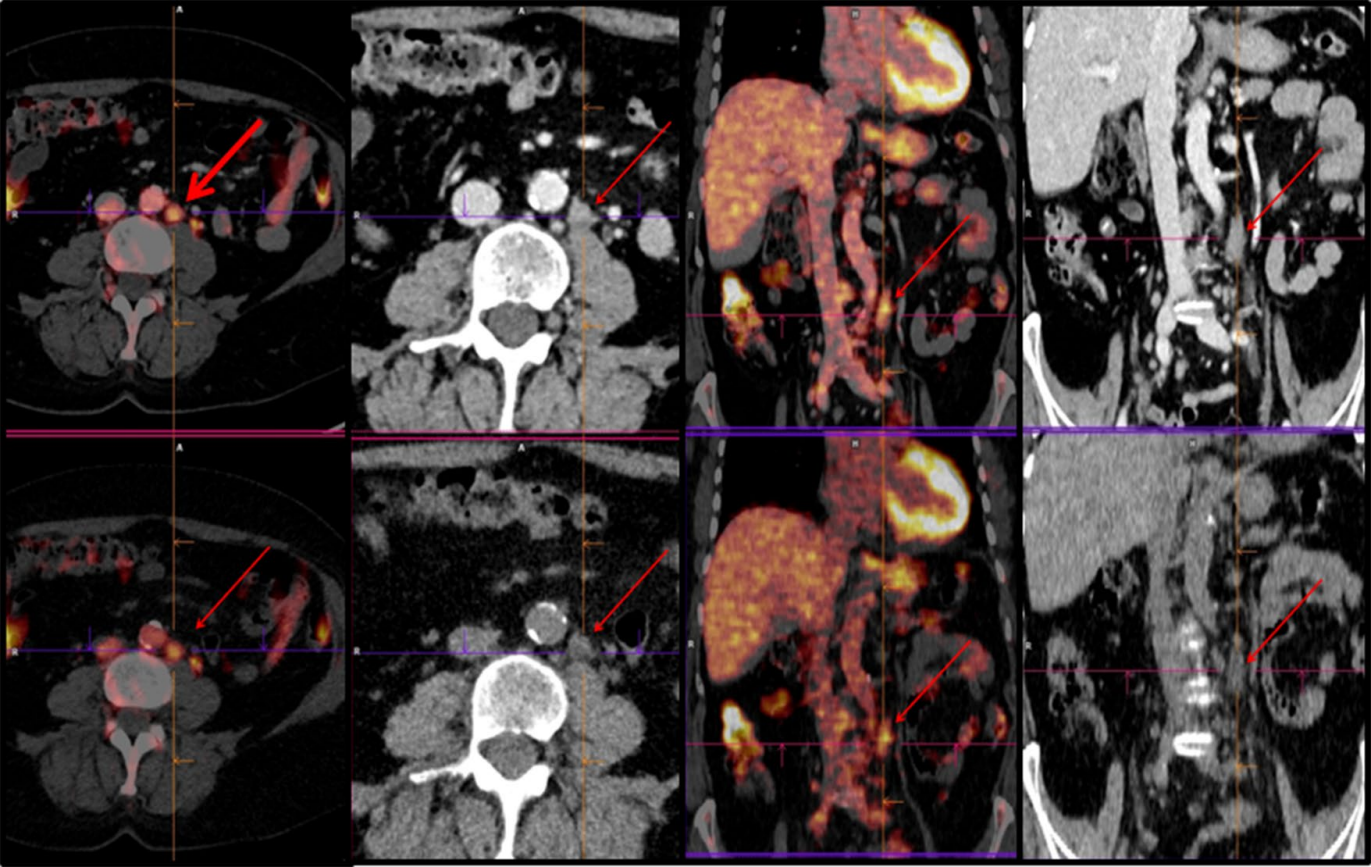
PET/ldCT showed the same number and sites of lesions highlighted by PET/ceCT [DS 5; SUVmax 8].
